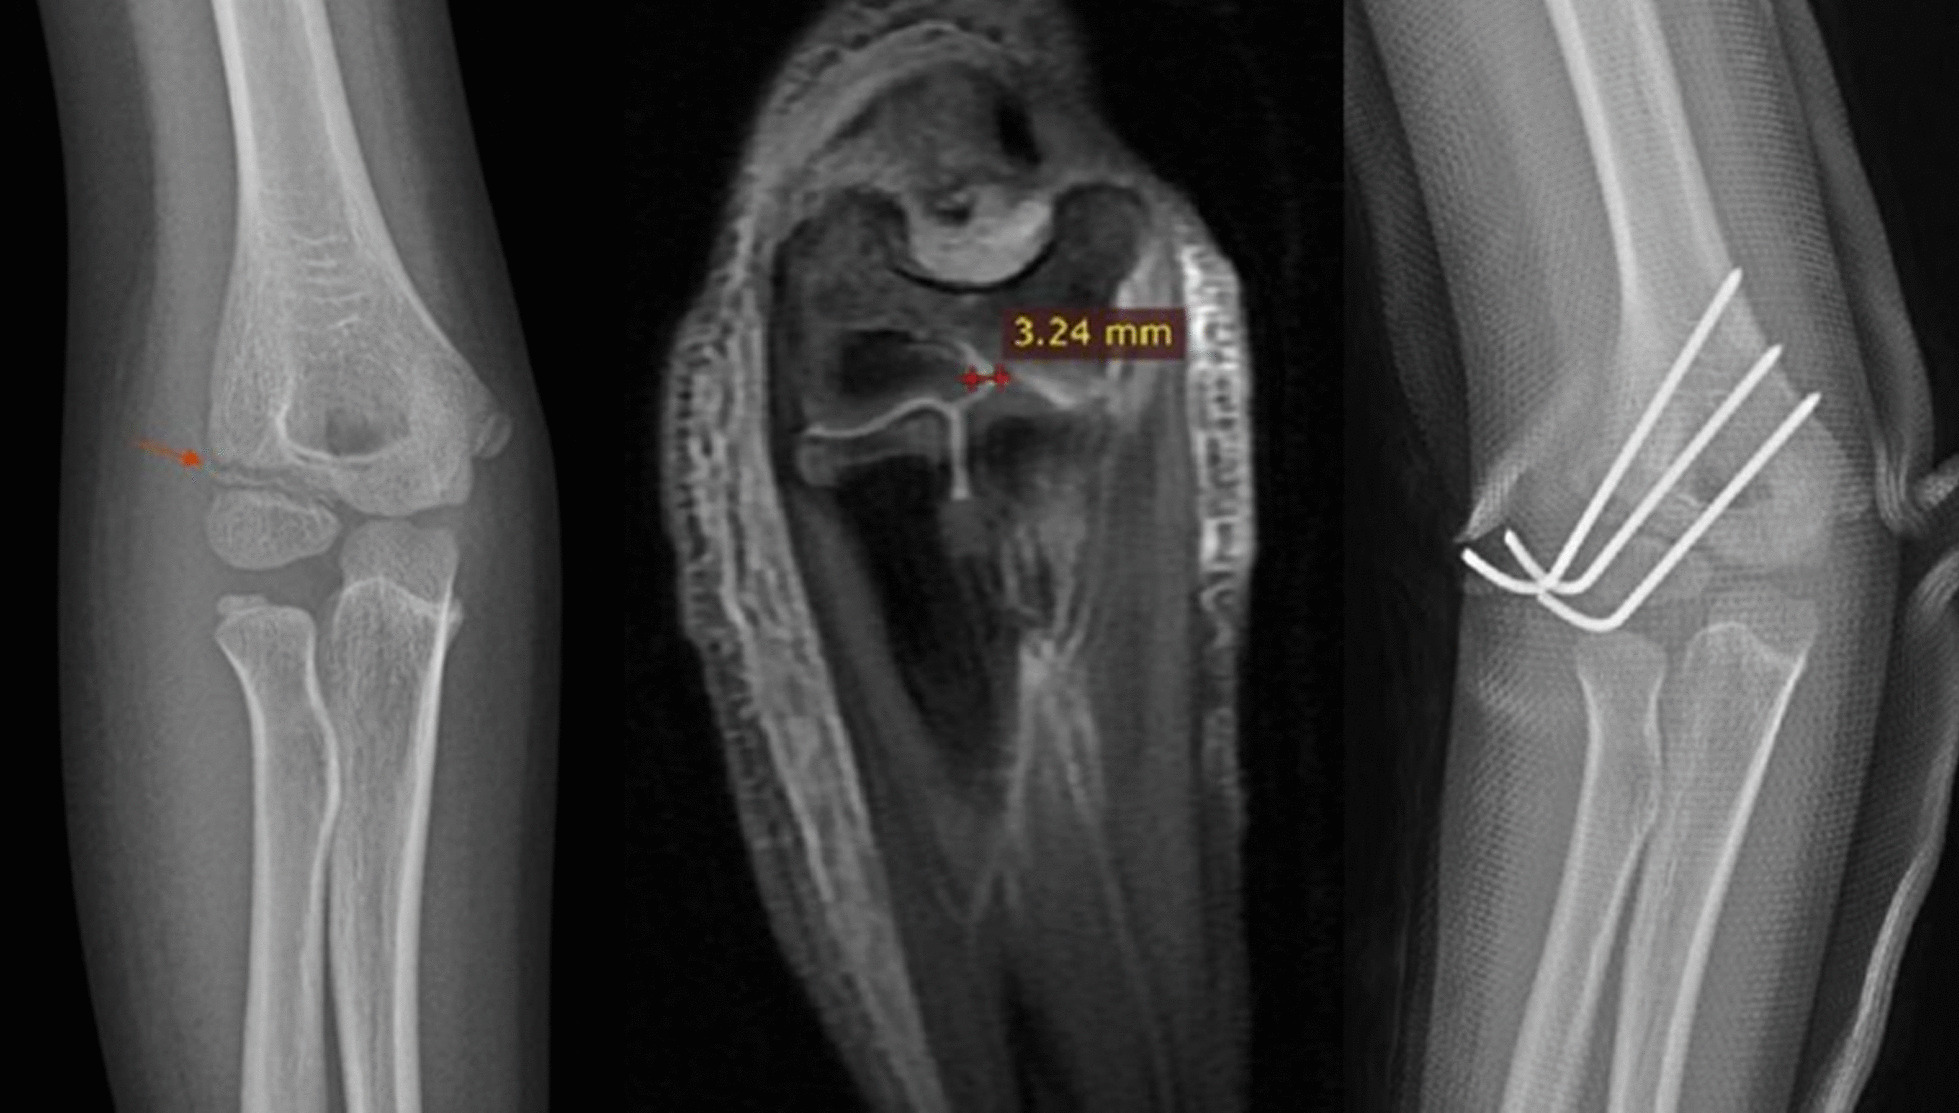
Eight years old boy with right elbow HLCF (Left: It showed slightly displaced fracture (LFS = 1.2 mm) by radiograph; Mid: MRI showed it was unstable fracture and FS-T2WI showed fracture line penetrated the humeral trochlear cartilage chain, and the displacement of articular cartilage was about 3.24 mm; Right: percutaneous K-wire fixation). HLCF, Humeral lateral condyle fracture; LFS, Lateral fracture space; PFS, Posterior fracture space
